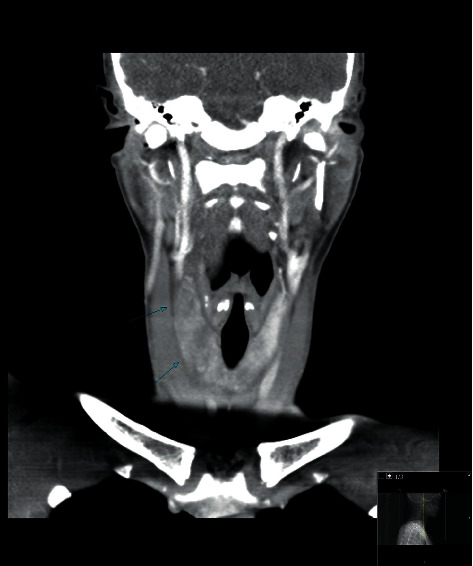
Contrast-enhanced CT image of head and neck, demonstrating thyroid enlargement and asymmetry. After the administration of contrast, the right lobe contained more hypodense areas without enhancement compared to the left lobe, as indicated by the arrows. There was no extravasation of the contrast medium. These findings are suspicious of contusion or laceration, though the possibility of autoimmune thyroiditis could not be excluded.
